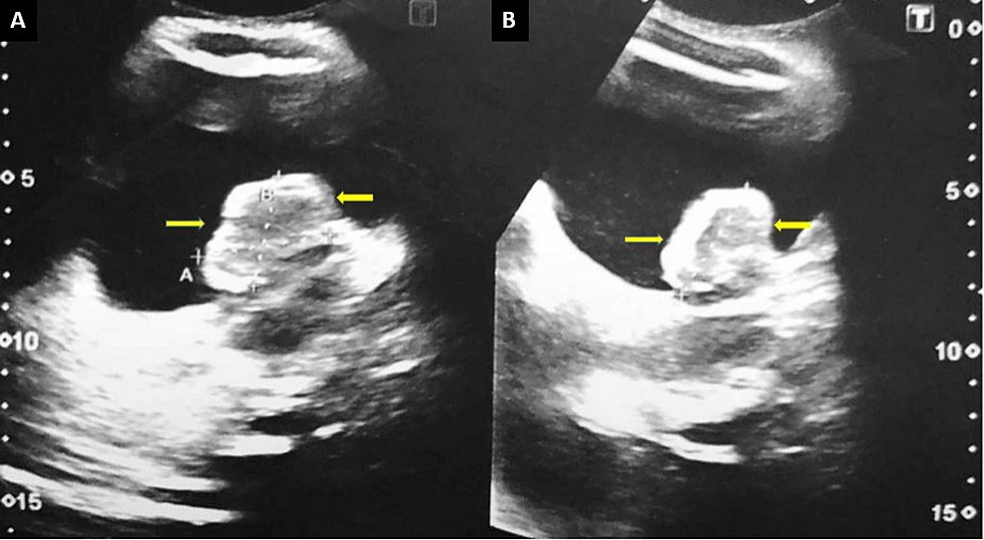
Ultrasound of the urinary bladder showing a large lobulated heterogeneously hypoechoic mass originating from the posterior wall.
